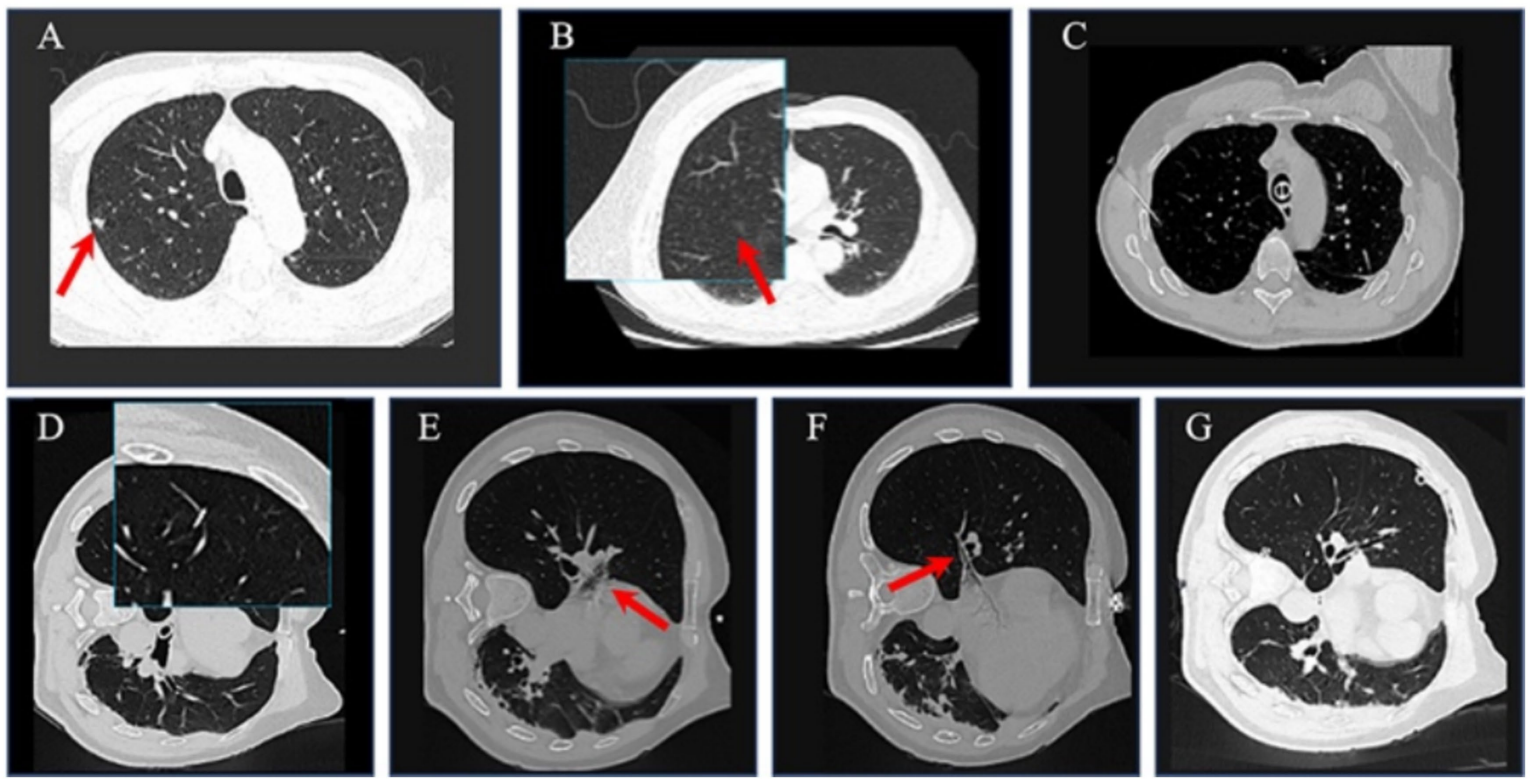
CT image of case 3. (A) Contrast-enhanced chest CT scan an 8 × 6 mm spiculated solid nodule (red arrow) with mild contrast enhancement in the apical segment of the right upper lobe. (B) Contrast-enhanced chest CT scan 5 × 4 mm ground-glass opacity nodule (red arrow) in the posterior segment of the right upper lobe. (C) Puncture of the right upper lobe apex. (D) Posterior branch of vein injured by puncture of ground glass tubercle of posterior upper lobe of right lung. (E) Right superior lobe venous gas embolism (red arrow). (F) Right lower lobe venous gas embolism (red arrow). (G) Postoperative whole chest CT scan showed disappearance of gas embolism in the right pulmonary vein [compared with (E,F) image during intraoperative puncture].
